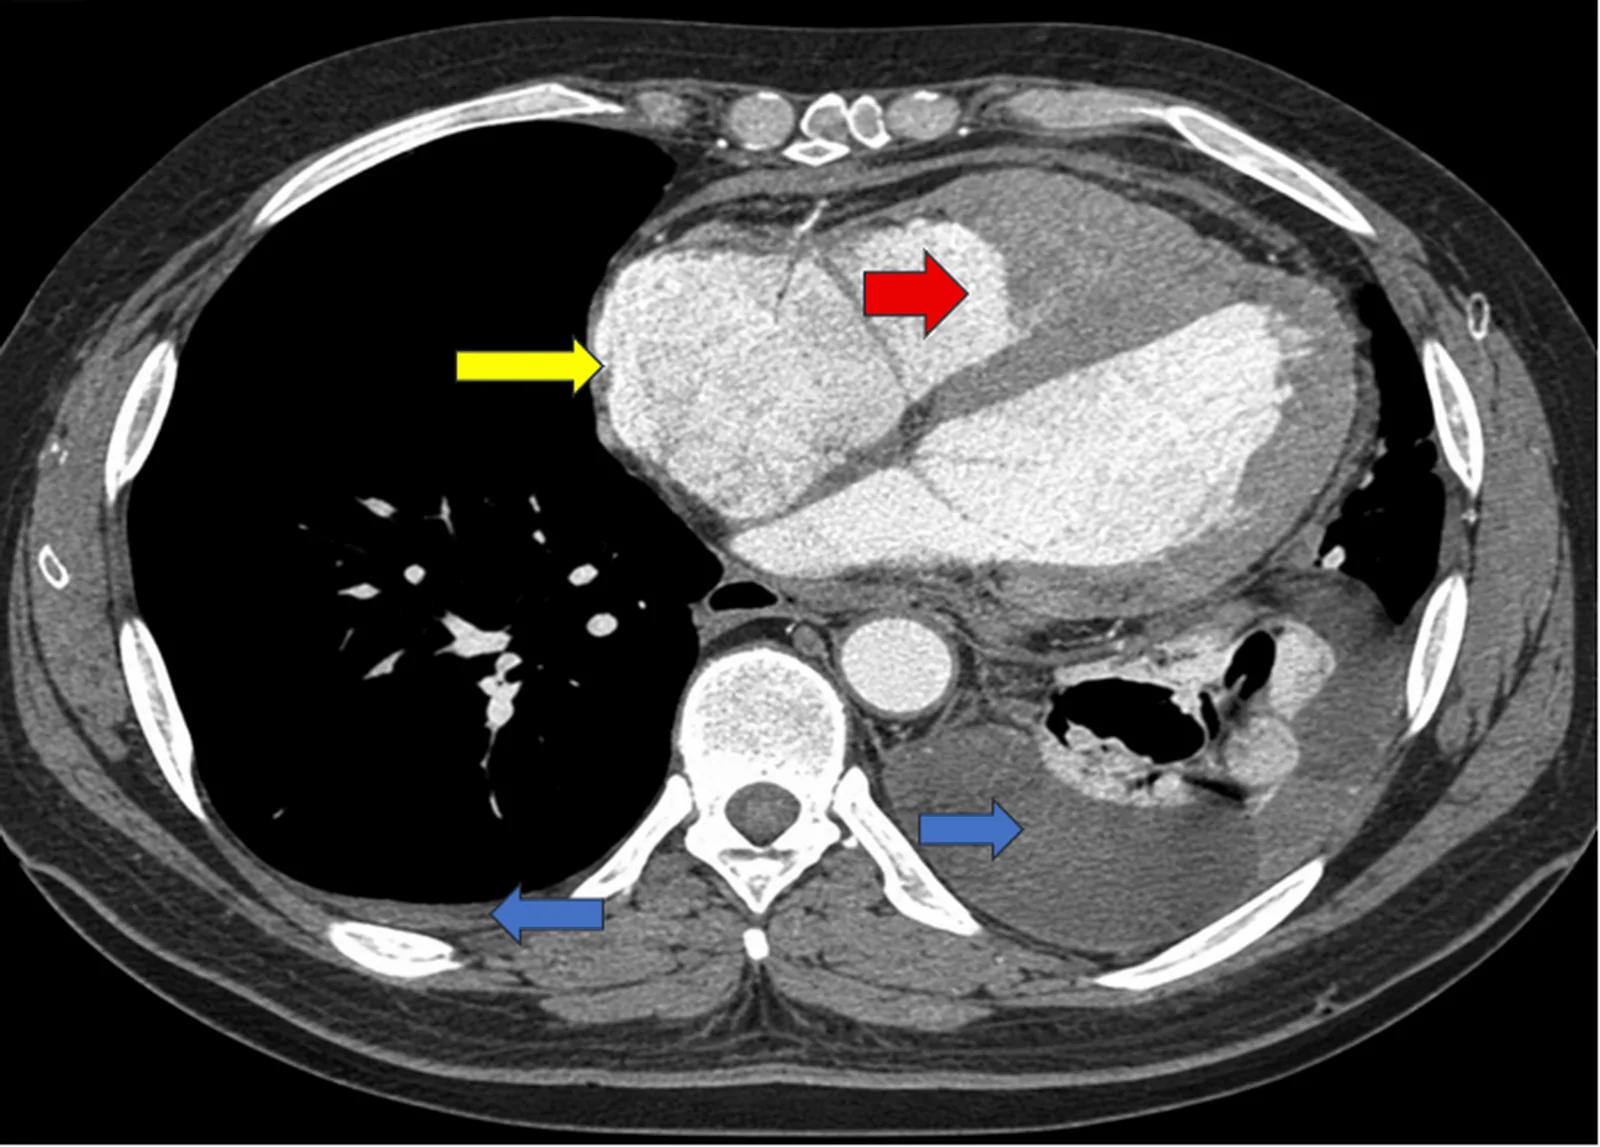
Contrast-enhanced CT of the chest. Axial contrast-enhanced CT image demonstrating a right ventricular intracavitary mass (red arrow) associated with right atrial dilatation (yellow arrow). Bilateral pleural effusions are also present (blue arrows), more prominent on the left side.
